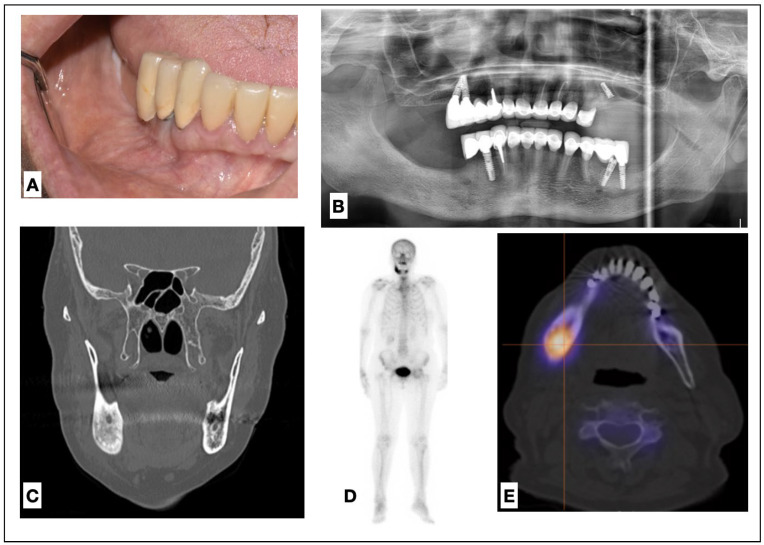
Intraoral view, no significant alteration can be noticed (A); dental panoramic radiograph, a slight increase in bone density of the right hemimandible can be noticed (B); CT scan in coronal view revealing endosteal sclerosis of the left hemimandible (C); total body scintigraphy showing a marked hyperuptake of the right mandible; no other area of involvement was found (D); axial view of scintigraphy with a marked hyperuptake of the left mandible (E).
